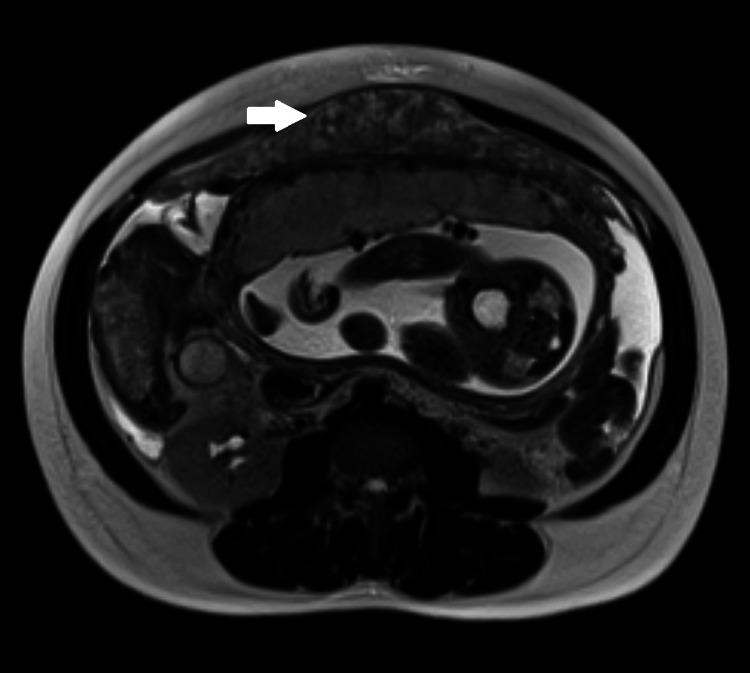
T2-weighted magnetic resonance imaging sequence of the abdomen of a pregnant woman from Haiti. An omental mass of intermediate intensity (white arrow) is shown anterior to the uterus.
